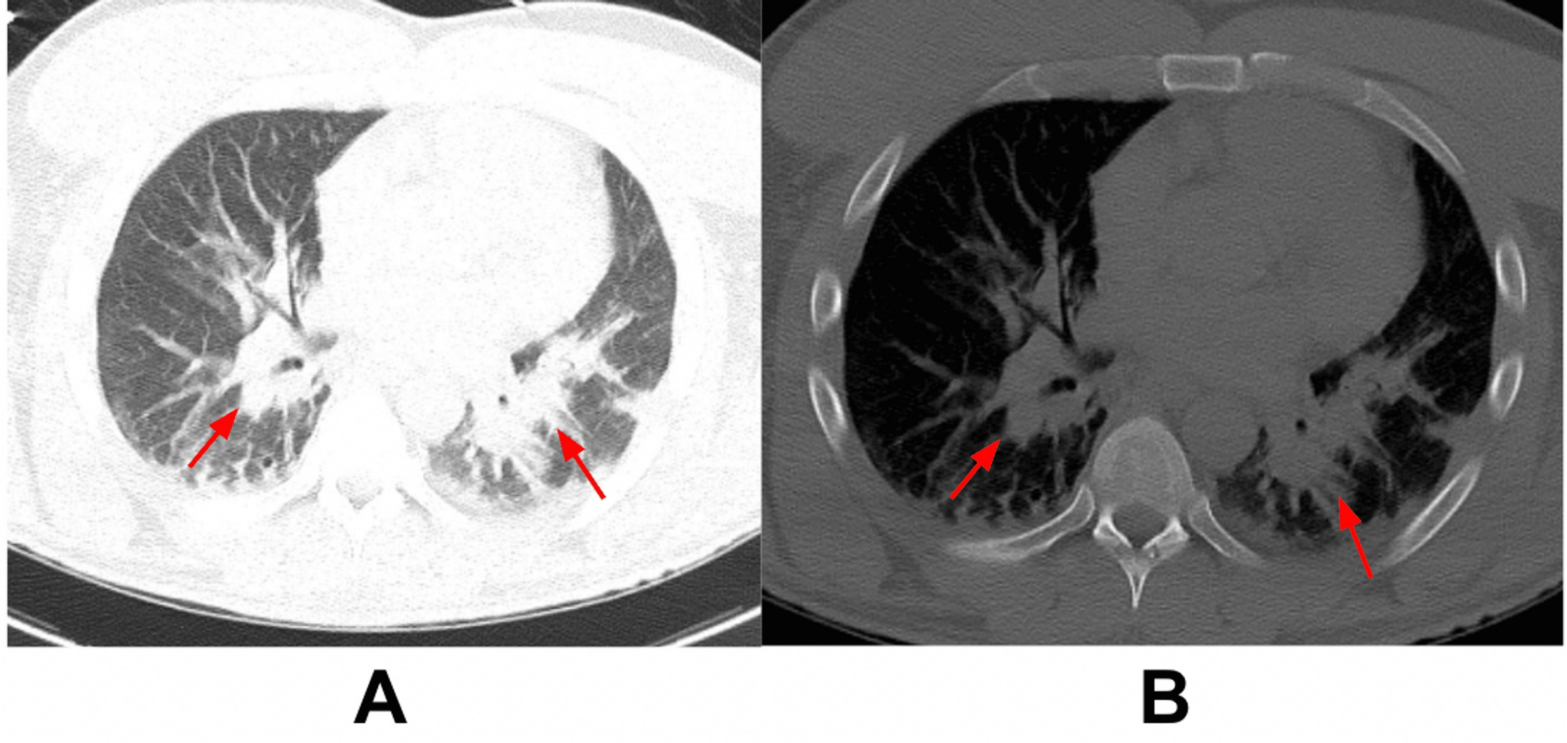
A and B show a CT chest without contrast depicting bilateral pneumonia, indicated by the red arrows.
